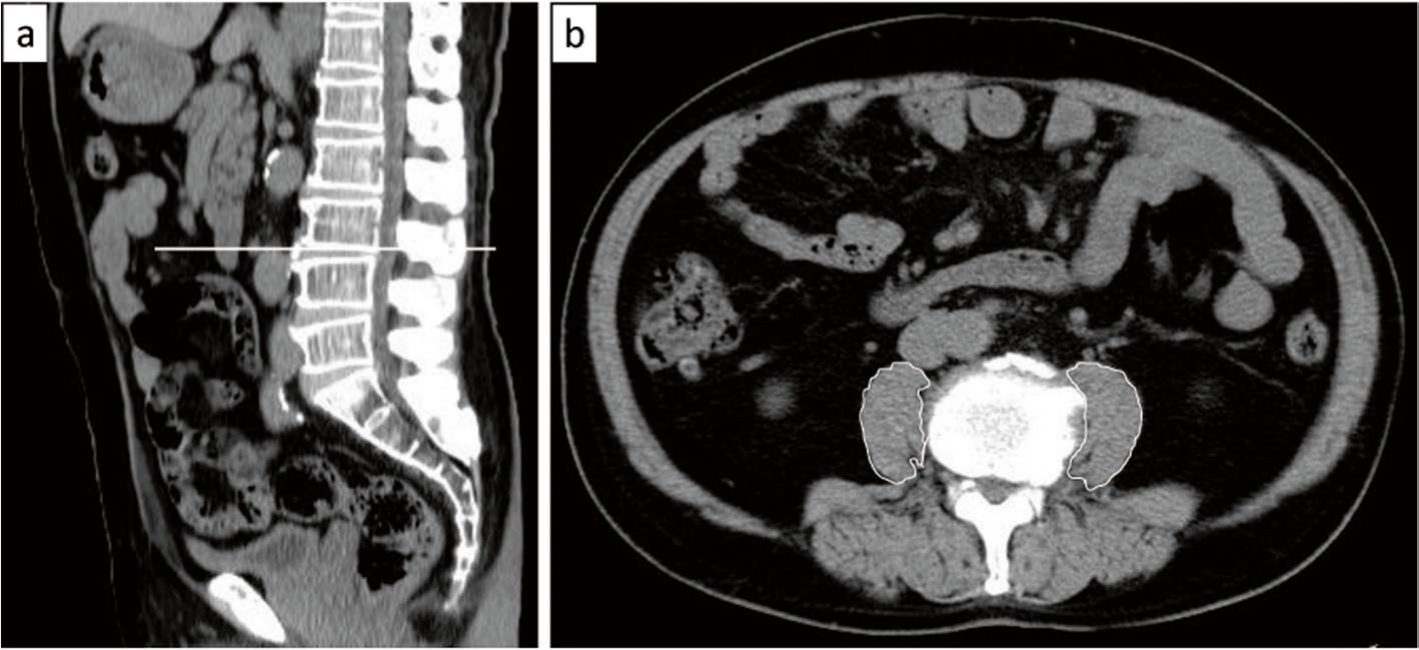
Measurement of psoas muscle area on the birth month’s CT scan. a. CT image showing the lower border of the third lumbar vertebra. b. The cross-sectional area of the bilateral psoas muscle was measured (manual trace method)
